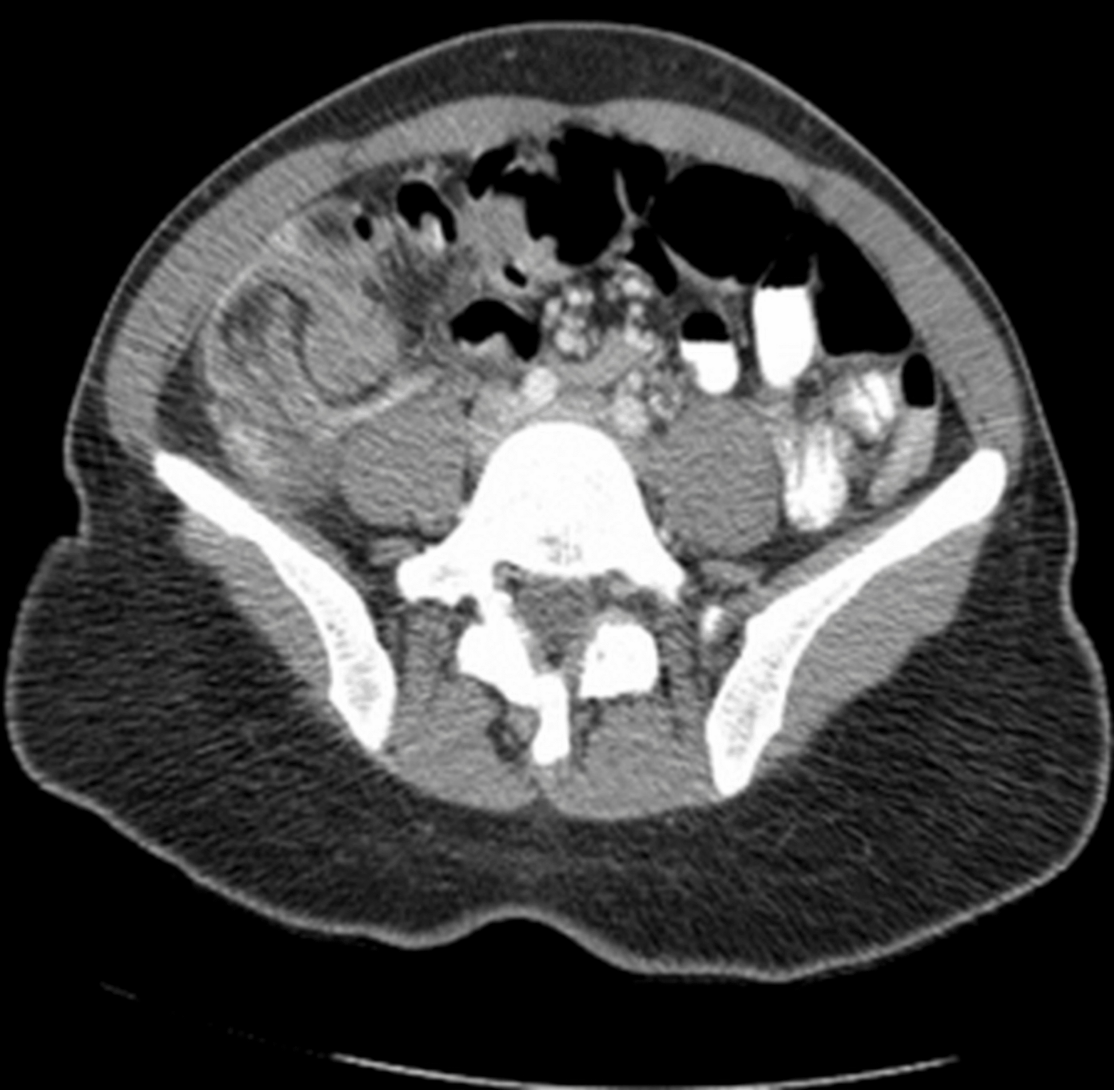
Computed tomography scan of the abdomen (axial view) showing the intussusception at the ileocecal junction.
